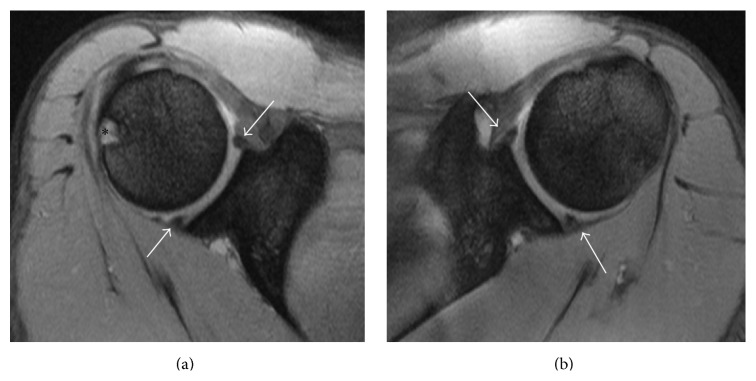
Axial DP FS SE MR images of the right (a) and left (b) shoulder (TR = 475; TE = 13.43; NEX = 1; EC = 1) showing normal labrum morphology bilaterally, without evidence of tears (white arrows). In (a) there is a cyst beneath the lateral humeral head margin, without pathologic significance (asterisk).
